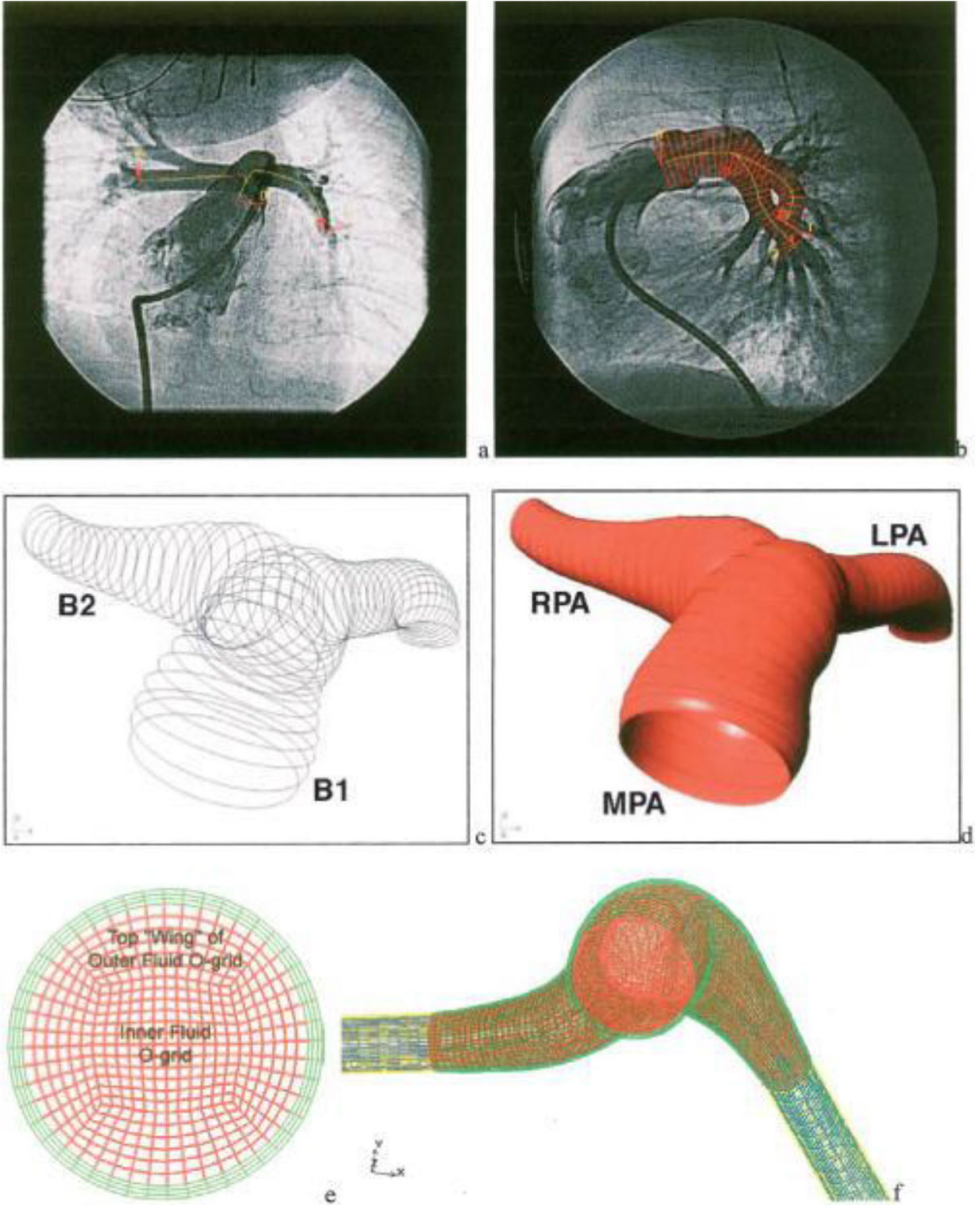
Patient-specific 3D geometry reconstruction and mesh generation procedures using X-ray angiogram. A) AP images of PA and B) lateral PA bi-plane angiogram showing centerlines overlapped on the AP image and diameter superimposed on the later image. C) the skeleton image of the PA, D) after creating surfaces on the skeleton image. E) The fluid domains (in red) and structure domains (in green) are shown, and F) a representative cross section of the MPA mesh [61].
